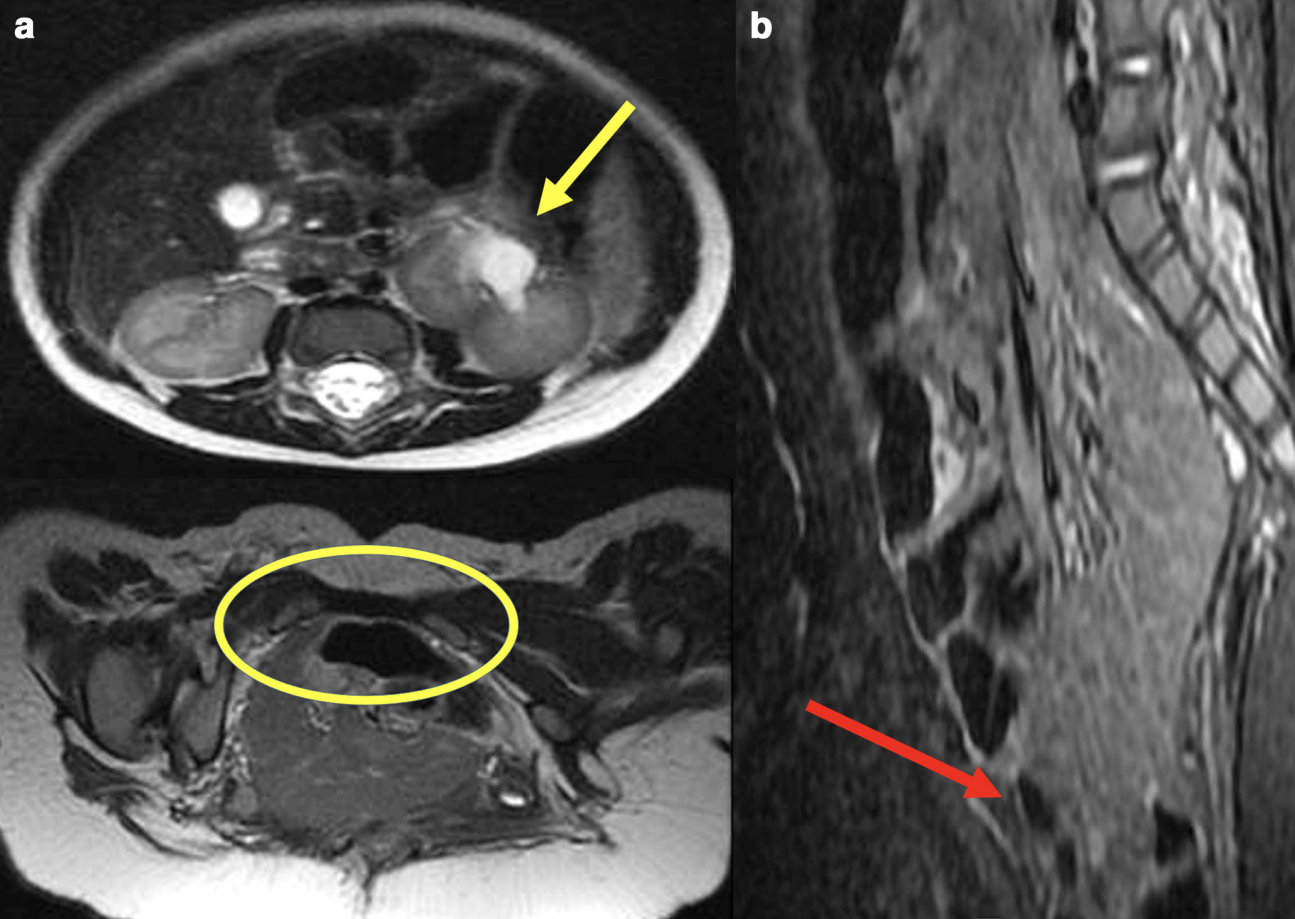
Abdominal MRI findings. Axial *T*_2_WI (a, b) imaging demonstrating the left kidney abnormal incomplete rotation with mild proximal pyelocaliceal distention (yellow arrow) and the pubic diastasis (yellow circle). Sagittal *T*_2_WI with fat suppression showing the small bowel loops inferior protrusion, contributing to the “prune-like” mass (red arrow). *T*_2_WI, *T*_2_weighted imaging
